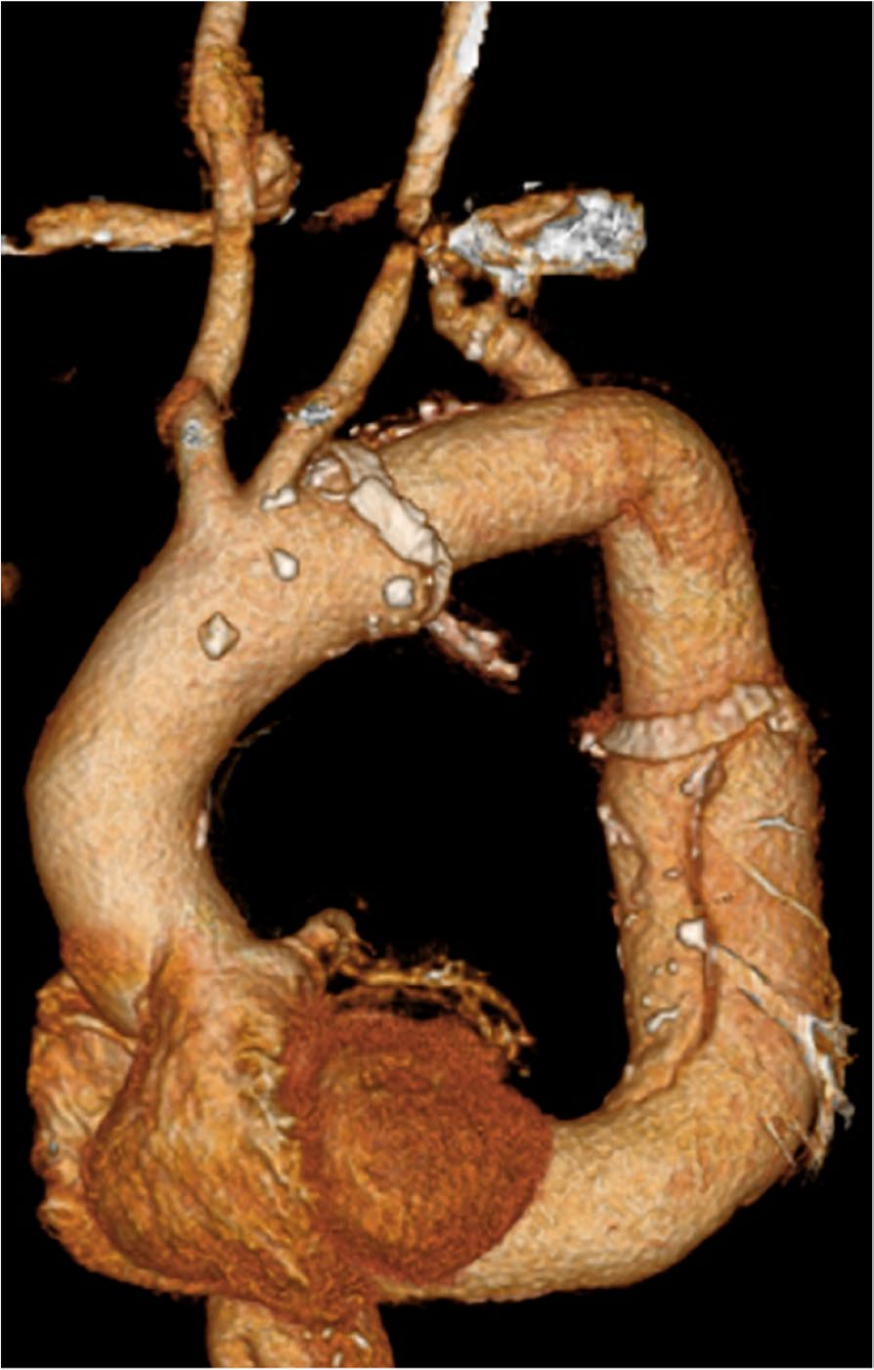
Postoperative computed tomography image after aberrant subclavian artery reconstruction and graft replacement
